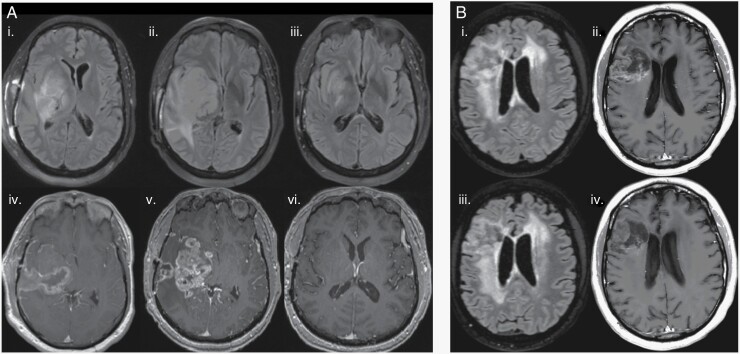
Treatment-related radiographic changes. (A) Pseudoprogression in a 50-year-old IDH wild-type diffuse midline glioma patient before initiating Pembrolizumab showing axial FLAIR (i) and T1 postcontrast (iv) images. Twenty days after Pembrolizumab therapy, there is an increase in vasogenic edema on axial FLAIR (ii) and contrast enhancement on T1 postcontrast (v) concerning for true progression versus pseudoprogression. Two months after Pembrolizumab therapy, there is a significant decrease in FLAIR hyperintensity (iii) and contrast enhancement (vi) confirming the previous diagnosis as pseudoprogression. (B) Pseudoresponse in a 55-year-old IDH wild-type glioblastoma patient before initiating bevacizumab (Avastin) showing axial FLAIR (i) and T1 postcontrast (ii) images. Two months after Avastin therapy, FLAIR hyperintensity and mass effect are unchanged (iii), but there was interval decrease in tumor enhancement on T1 postcontrast (iv).
